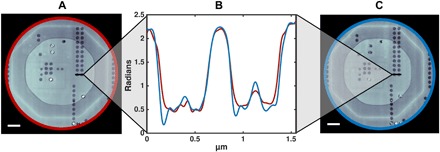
Confirmation of quantitativeness. (A and C) A reconstruction of the same ASIC, measured with Fourier ptychography (A) and conventional ptychography (C) (*16*). Scale bar, 2 μm. Resolution estimate for (A) is 47 nm and that for (C) is 41 nm (*16*). (B) The phase of the x-ray Fourier ptychographic reconstruction was compared to its conventional ptychographic counterpart. (C) The line cuts of both reconstructed phases reveal that the phase profiles match and thus a quantitative reconstruction can be provided (red for the Fourier ptychographic reconstruction and blue for “conventional” ptychography).
